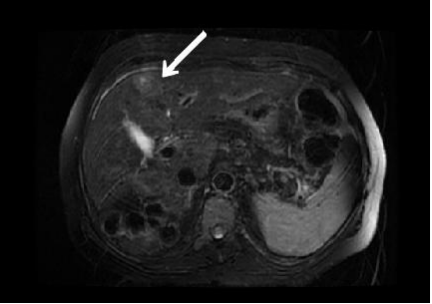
Magnetic resonance imaging scan of the abdomen with a hepatocellular carcinoma indicated by the arrow.
